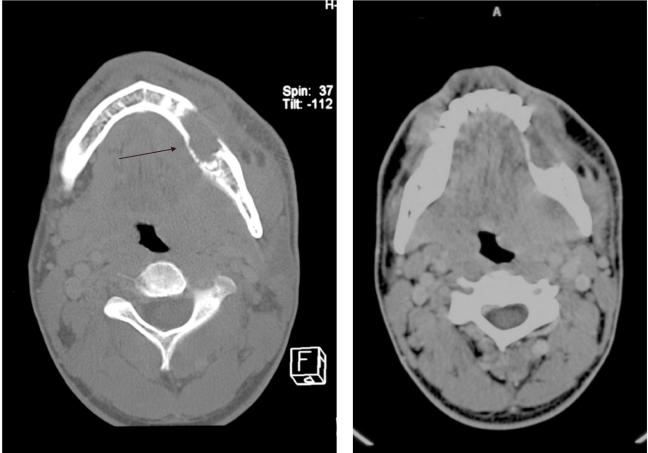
CT scan at level of body of the mandible (left: bone WW: 2344, WC: 440 and right: soft tissue window WW: 250, WC: 50) showing lytic destruction (arrow in left figure) with associated soft tissue swelling. Enlarged bilateral anterior cervical nodes noted.
